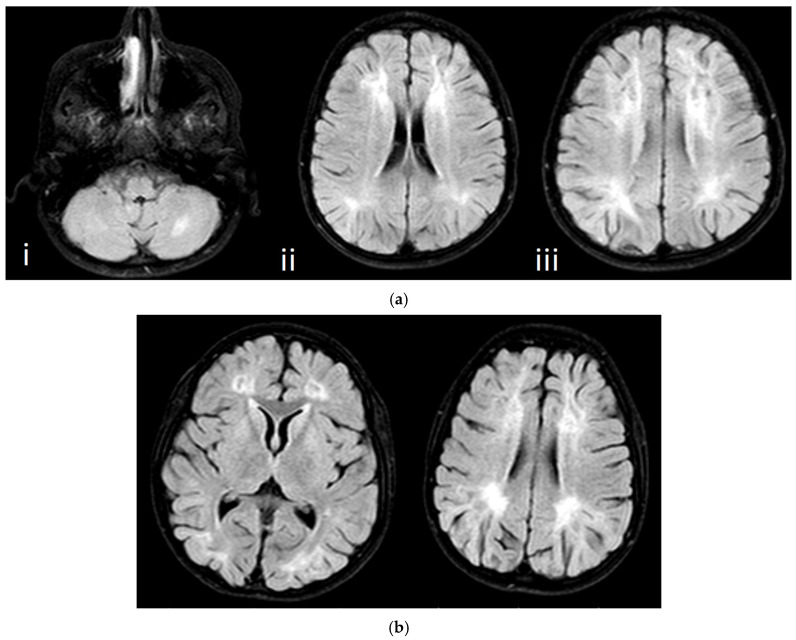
MRI images: (a) Case 4a—i. a 13 × 6 mm hyperintense leukomalacic area in the left cerebellum in the fluid-attenuated inversion recovery (FLAIR) sequence, ii. symmetrical hyperintense leukomalacic areas in periventricular deep white matter in the vicinity of the anterior and posterior horns of both lateral ventricles in the FLAIR sequence in cranial MRI examination, iii. symmetrical hyperintense leukomalacic areas in centrum semiovale the FLAIR sequence in cranial MRI examination, and (b) Case 4b—symmetrical hyperintense leukomalacic areas in periventricular deep white matter in the vicinity of the anterior and posterior horns of both lateral ventricles in the FLAIR sequence in cranial MRI examination.
